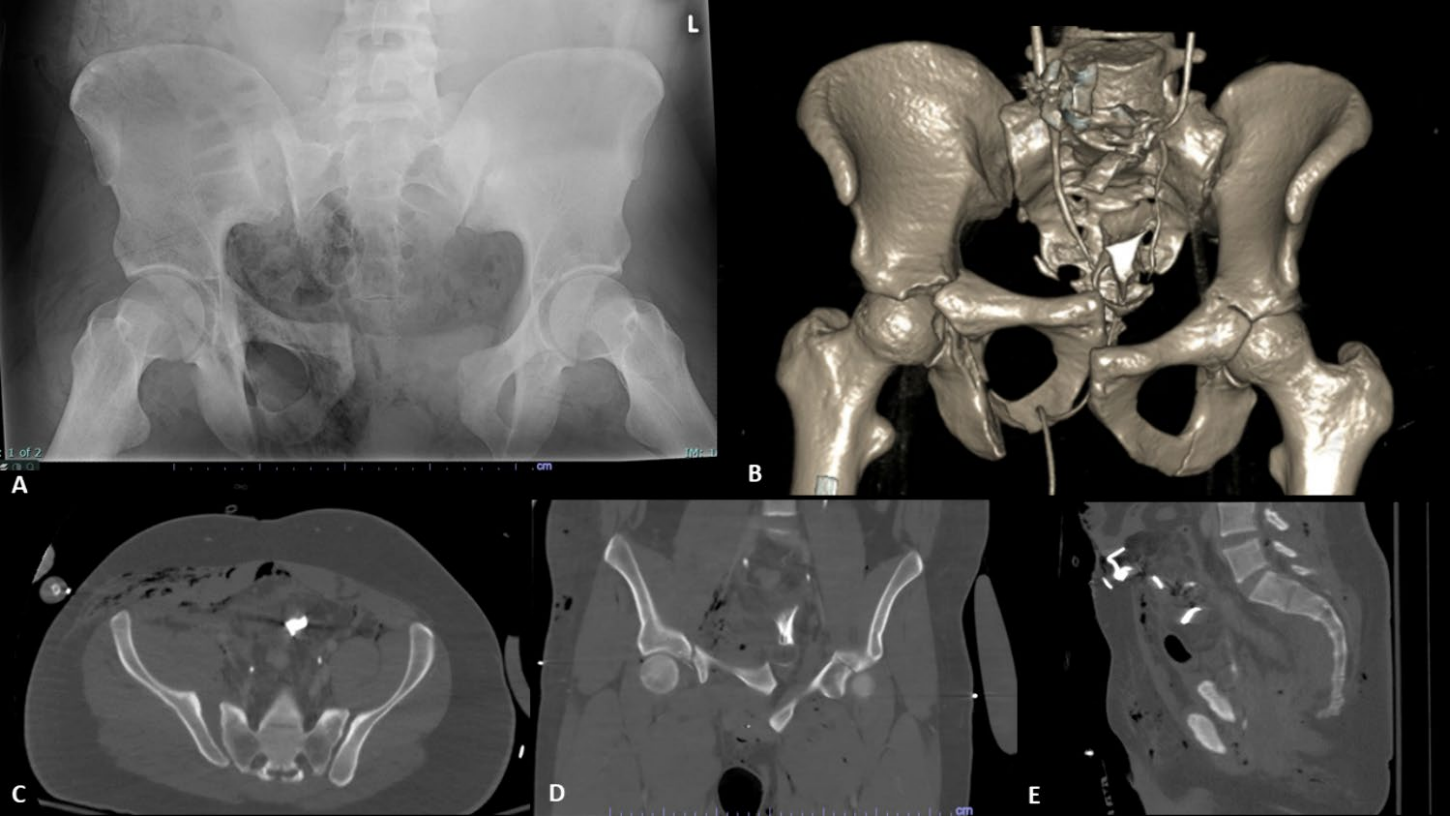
A Initial AP pelvis radiograph per ATLS protocol without circumferential pelvic compression. B 3D surface reconstruction and C axial, D coronal, E sagittal representative images of pelvic CT scan after application of circumferential pelvic compression
